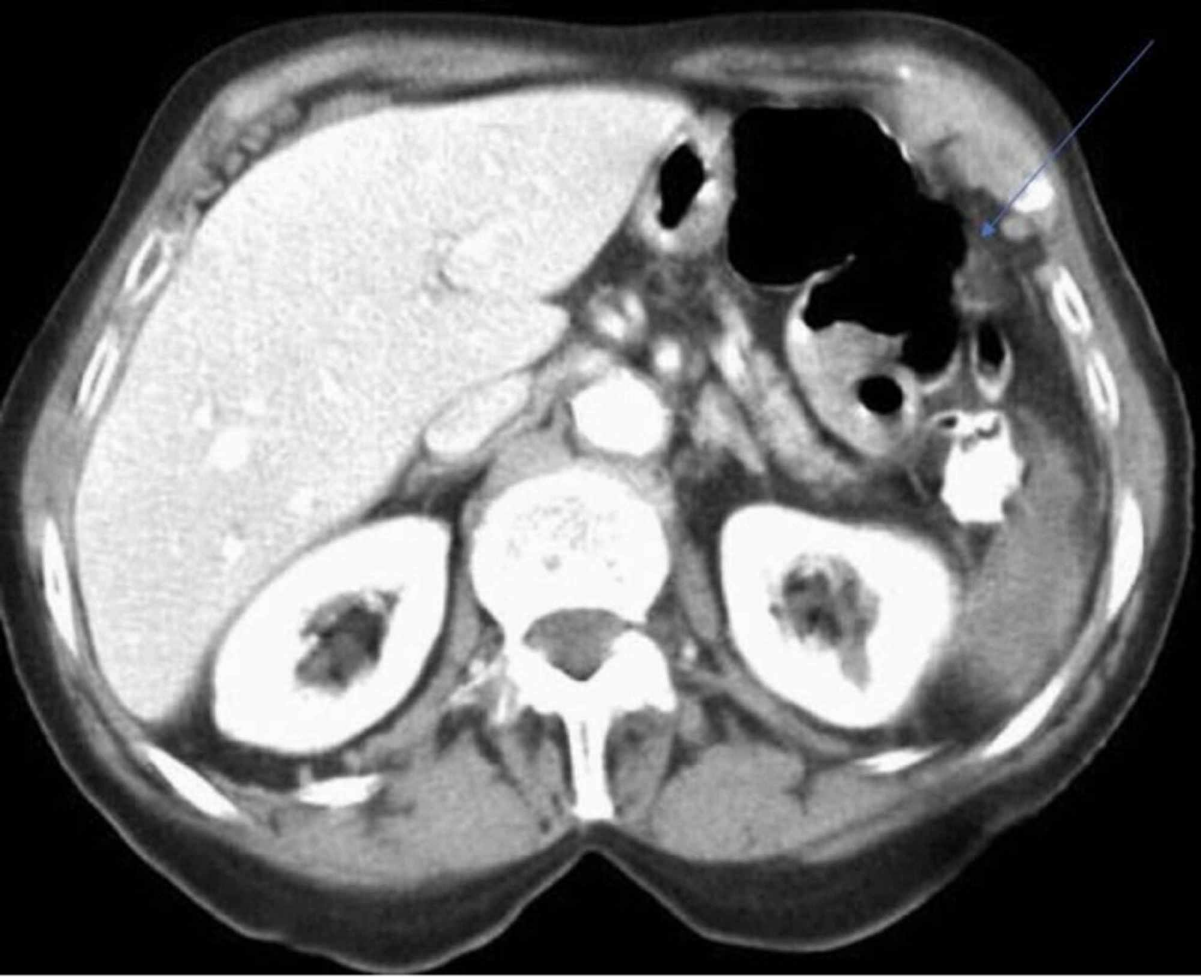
Abdominal CT scan frame showing the splenic injury caused during the colonoscopy: grade II splenic laceration without signs of hemoperitoneum; anterior capsule of the spleen was densely adherent to the splenic flexure of the colon and partially avulsed.
